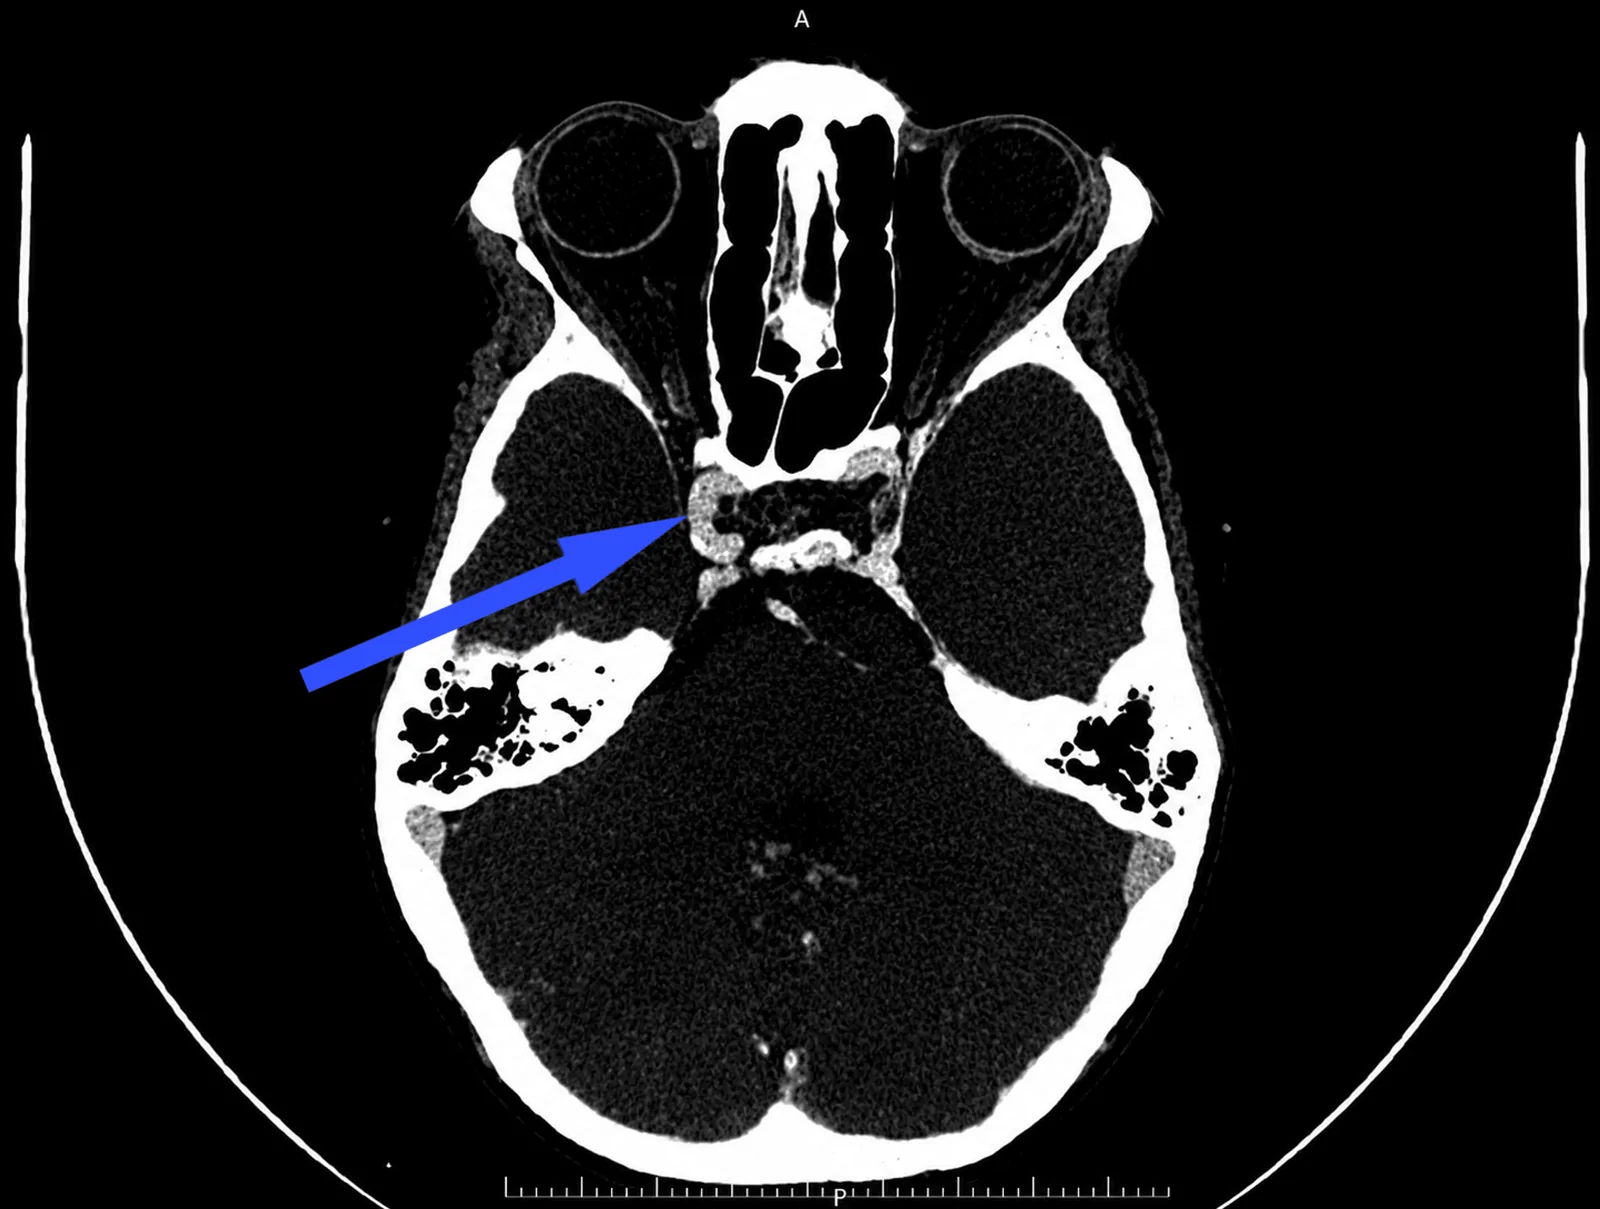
Carotid and cerebral computed tomographic angiography Thick-walled calcification in the cavernous segment of the right internal carotid artery (blue arrow).
